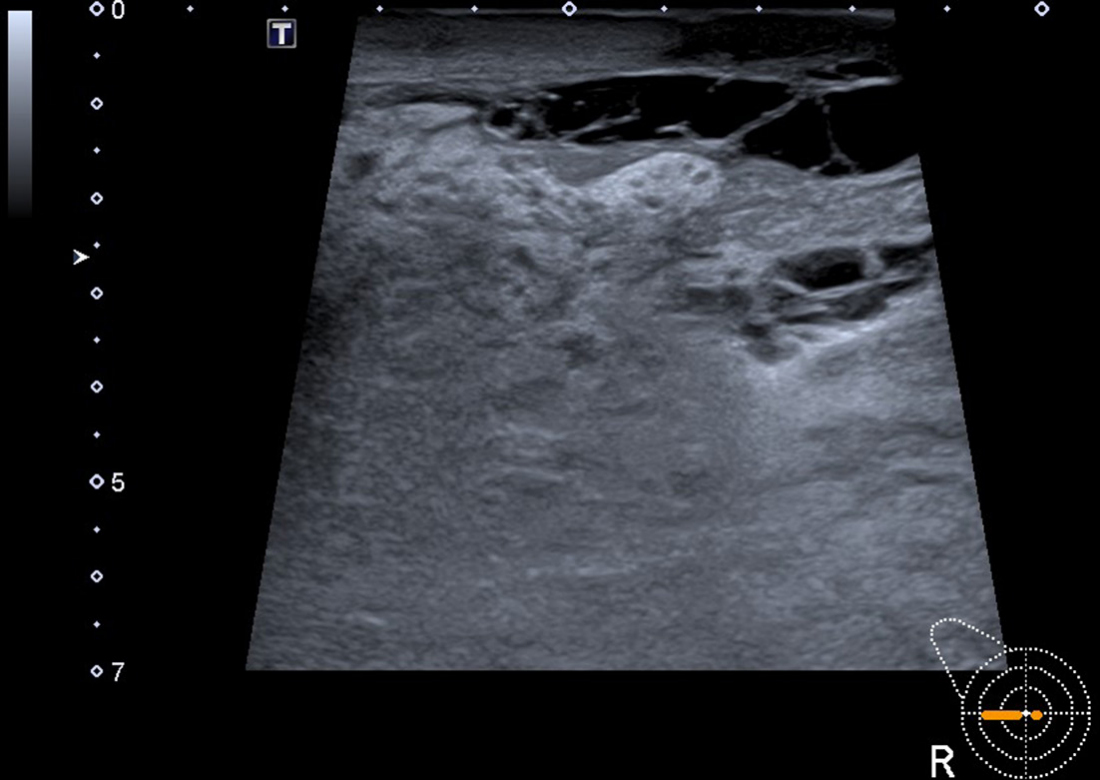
Grayscale ultrasound image of the right breast displaying skin thickening and anechoic subcutaneous structures with thin septations that could be dilated lymphatic ducts or cystic changes, but that were most likely veins on Doppler mode.
